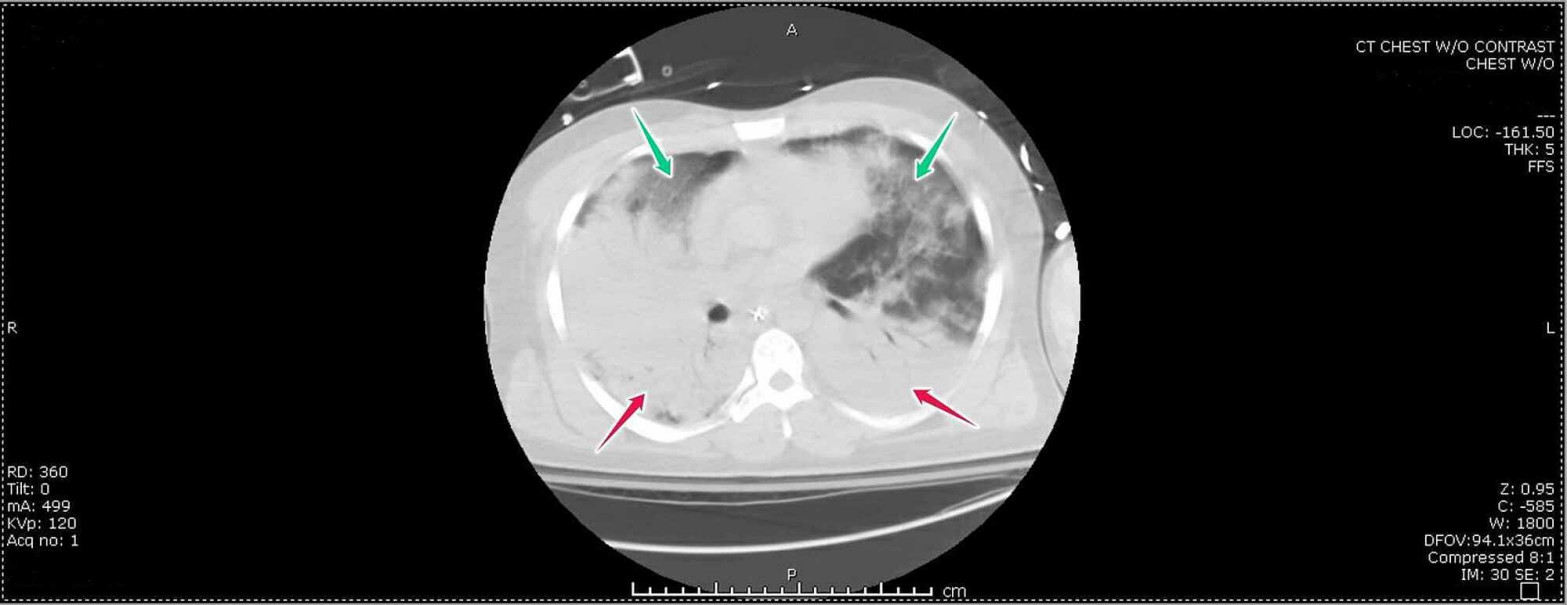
CT scan of the chest showing dense bilateral opacification of the mid and lower lung segments (red arrows) and ground-glass opacities in mid and upper segments bilaterally (green arrows).
